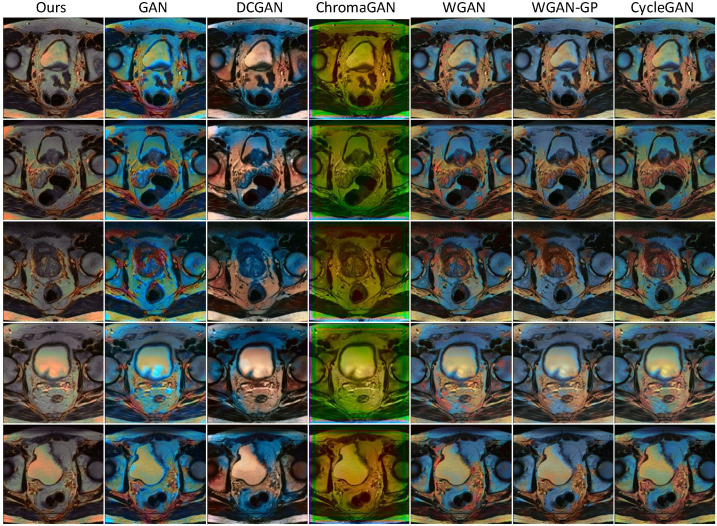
Qualitative colorization results of the prostate T2-weighted MRI using different image colorization approaches.
